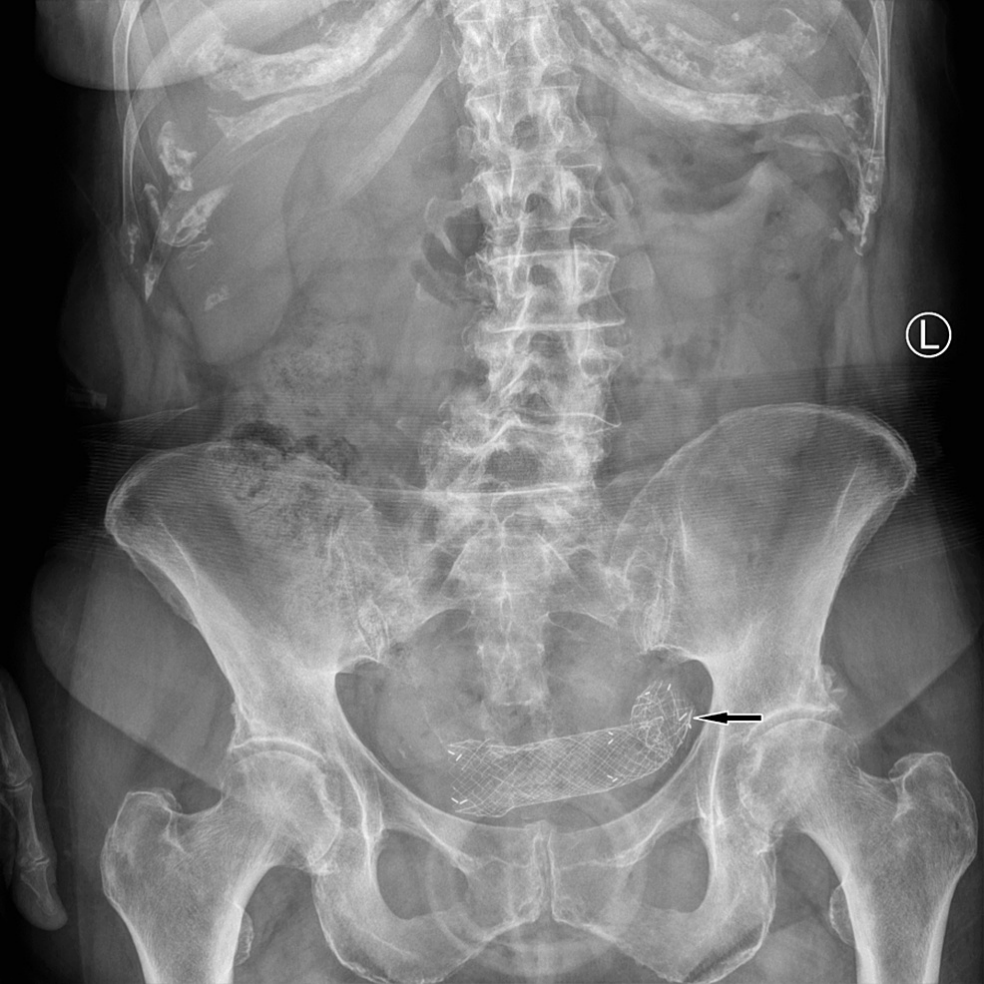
Plain film of the abdomen demonstrating the migrated stent (black arrow) located within the lower abdomen.
